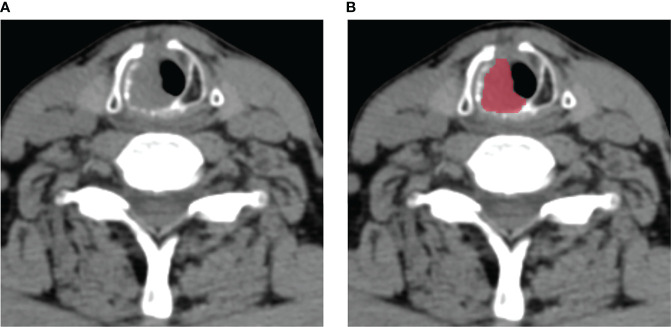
An example of manual segmentation in laryngeal squamous cell carcinoma (LSCC). (A) Localized space-occupying lesion of LSCC was observed on plain CT image; (B) Manual segmentation on the same axial slice was depicted with red label.
